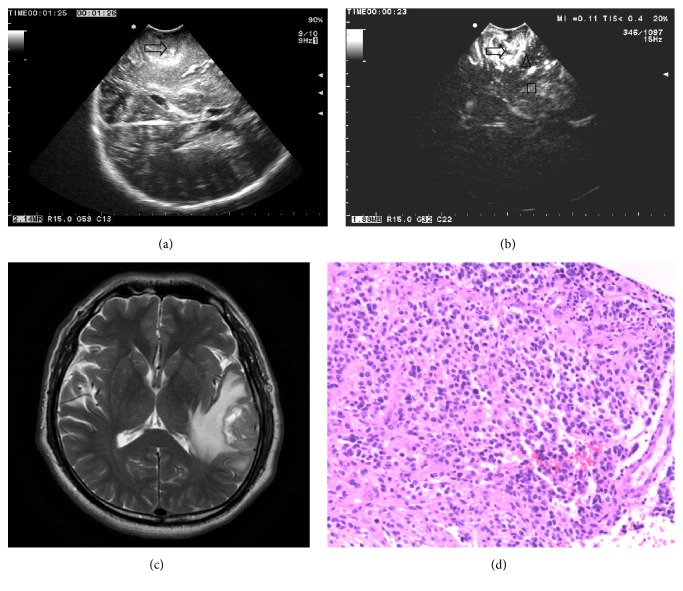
(a) Ultrasound image of high grade glioma shows that the tumor is hyperechoic, and the boundary is not clear and heterogeneous internal echo. The edema is obvious (arrow tumor). (b) CEUS shows that the echo of the glioma is enhanced significantly, brain edema is enhanced lower than the glioma, and brain tissue is enhanced lower than the glioma and edema (arrow showed the glioma, brain edema triangle shown, and the box showed normal brain tissue). (c) MRI T2W shows that the high grade glioma is mixed-signal, and there is edema around it. (d) The pathological image of the same patient shows that the microvessel is abundant (HE ×100).
